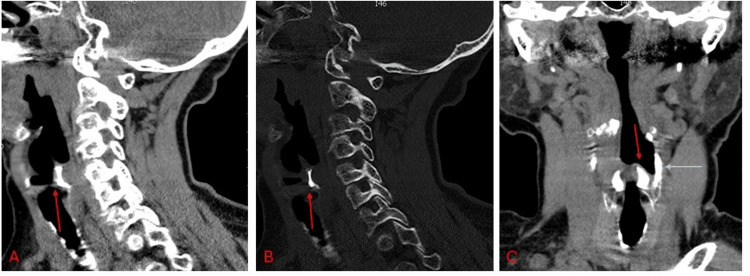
Arytenoid cartilage ossification with minimal involvement of the laryngeal skeleton Constructed CT of the neck, sagittal plane, shows complete ossification of the arytenoid cartilage, excluding the vocal process. A. with soft tissue density (red arrow) and B. with bone density (red arrow). C. Constructed CT of the neck, coronal plane, with soft tissue density, shows complete ossification of the arytenoid cartilages (red arrow), ossification of the superior thyroid horns (grey arrow), and no sign of cricoid involvement, highlighting the ossification at the tip of the arytenoids.
